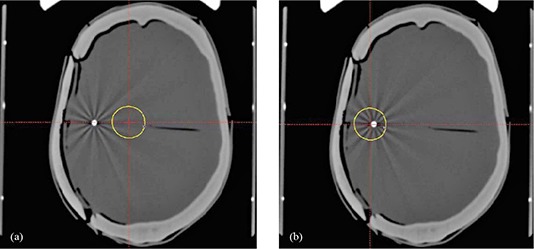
Treatment planning axial CT slices through intact skull for 5.5 cm depth (a) and 2.5 cm depth (b).
